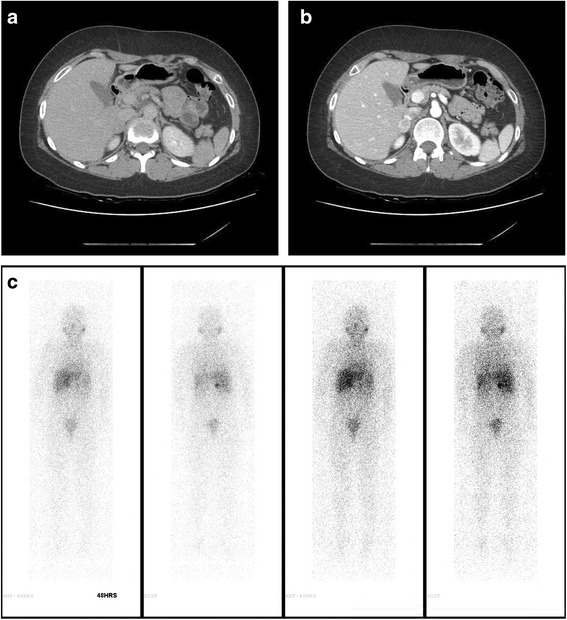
(a) Abdomen computed tomography axial image with contrast enhancement shows a well-defined mass measuring 2 cm in right adrenal gland. Hounsfield unit (HU) at pre-contrast enhancement image is 42 HU, 138 HU at 1 min delayed image. (b) 10 min delayed image show a 75 HU and washout ratio is 65%. (c) I-131 metaiodobenzylguanidine (MIBG) image show a mass with increased uptake in right adrenal gland
